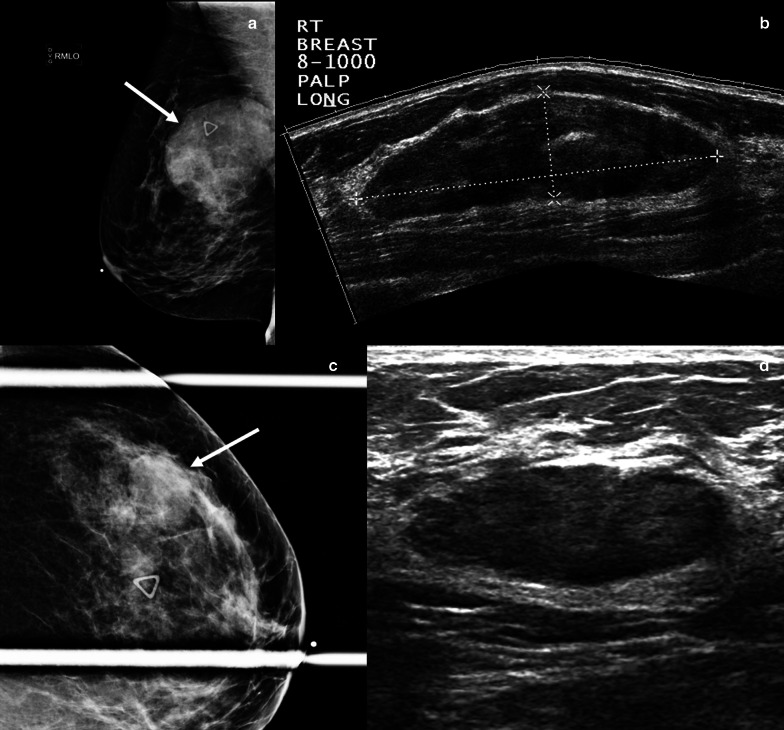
Pseudoangiomatous stromal hyperplasia. A 41-year-old woman presented with a palpable breast mass. Mediolateral oblique mammogram (a) shows a high-density well-circumscribed mass (arrow). Longitudinal grayscale ultrasound (b) shows a large, oval circumscribed mass. Core needle biopsy showed pseudoangiomatous stromal hyperplasia (PASH). A different patient, a 40-year-old woman, was found to have a new breast mass (arrow) on mammography, craniocaudal spot view (c). Transverse grayscale (d) ultrasound reveals a corresponding oval isoechoic circumscribed mass. Core needle biopsy showed PASH
